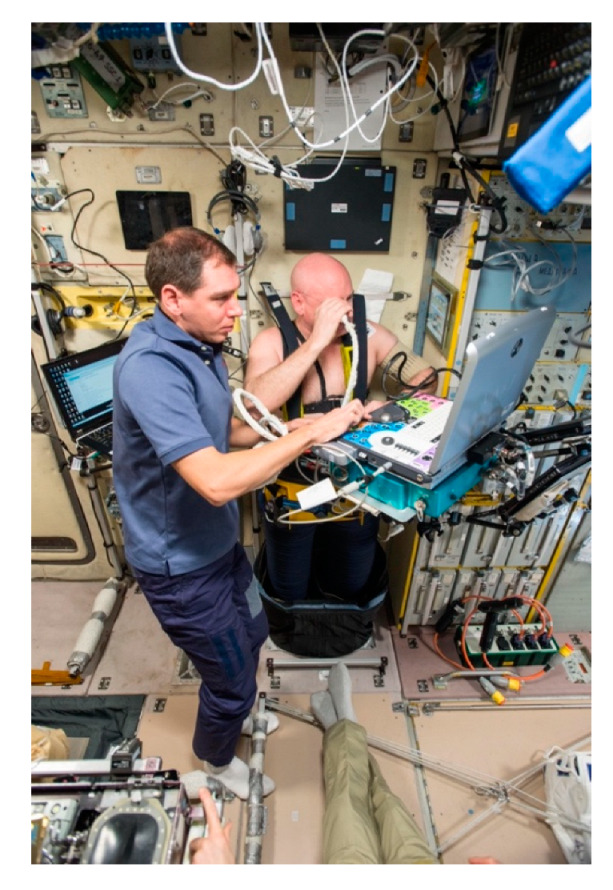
Ultrasound onboard the ISS for measuring fluid shifts. Ultrasound for fluid shift experiments performed on NASA astronaut Scott Kelly (NASA Image: ISS045E015549).
